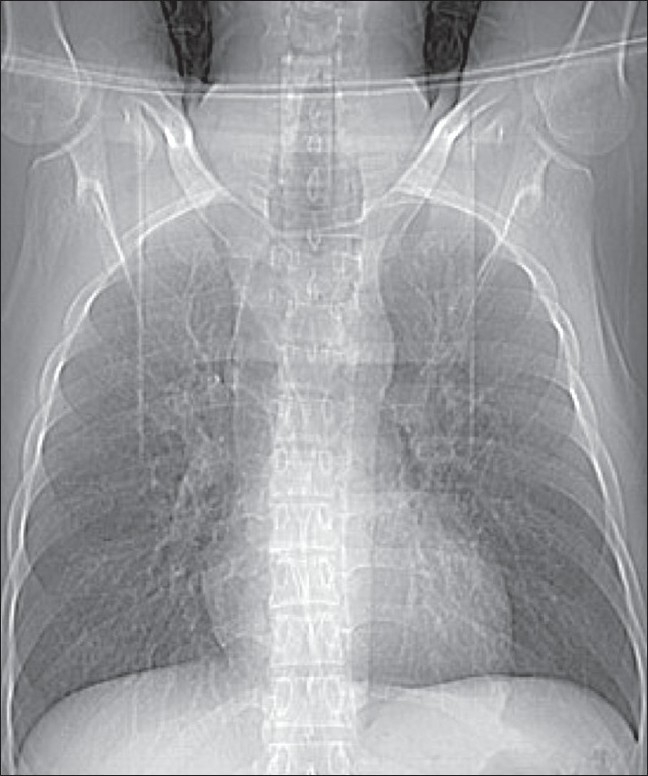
CT scannogram shows enlargement of the trachea and bronchi and bilateral bronchiectasis
